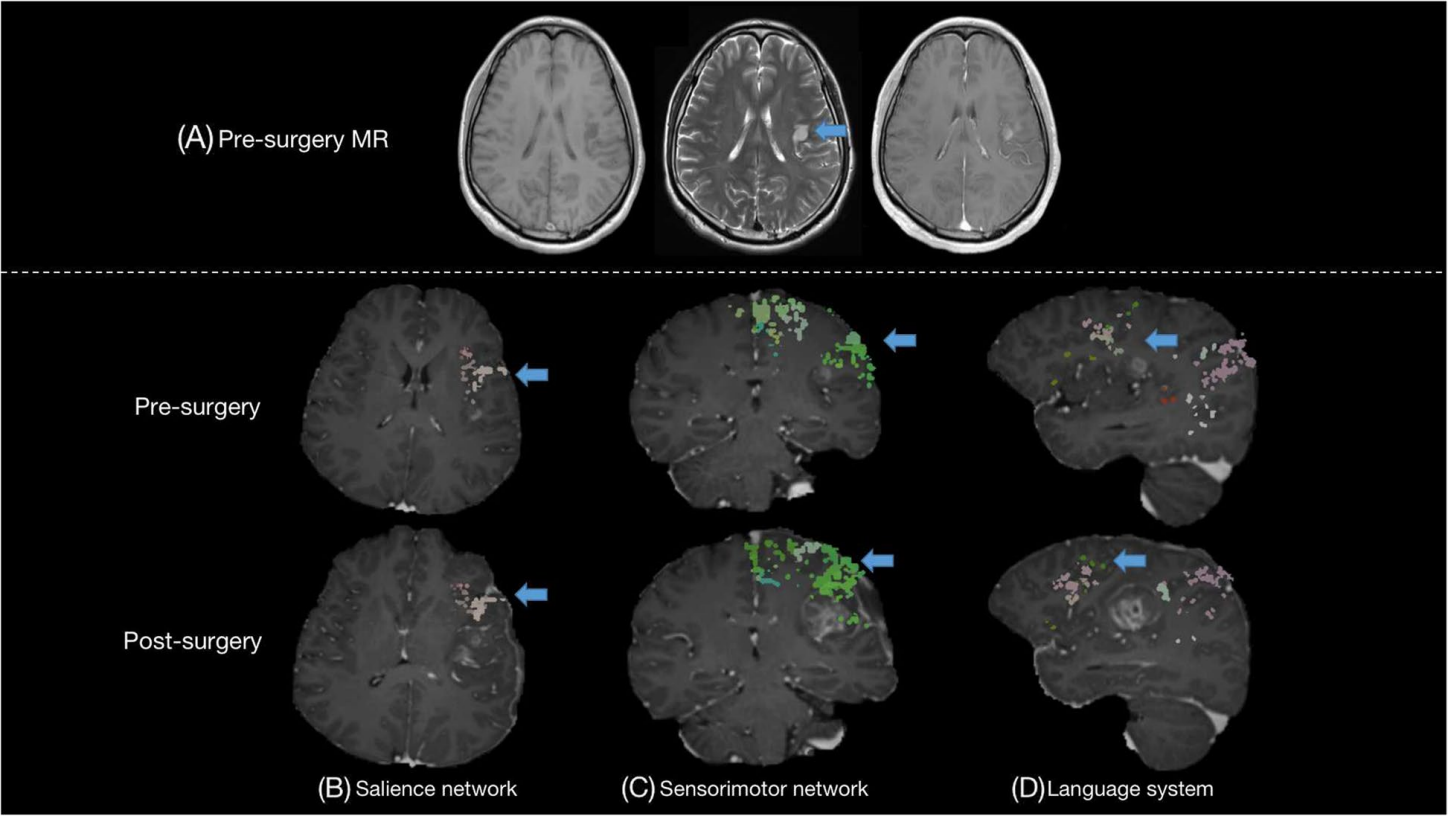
MRI images and the networks showed by the Quicktome in patient no. 1, who had the glioma resection in the left temporal and insula lobe. A T1, T2, and postcontrast T1 images before surgery; the Quicktome software demonstrates part of the B salience network, C sensorimotor network, and D language system, where were close to the tumor before surgery and the resection location after surgery
